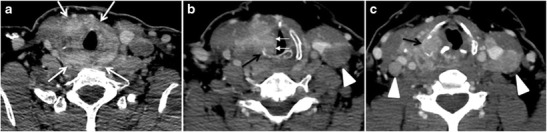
A 61-year-old female patient with locally aggressive PTC. a Enhanced axial CT scan of the neck demonstrates a heterogeneous infiltrative thyroid mass. This mass diffusely involves the entire gland and circumferentially encases the trachea with involvement of bilateral tracheoesophageal grooves (*white arrows*). b, c Additional axial cranial images show right cricoid cartilage destruction (*black arrows* in b), right thyroid cartilage destruction (*black arrow* in c), right vocal cord paralysis (*white arrows* in b), and bilateral cervical lymphadenopathy (*arrowheads*)
